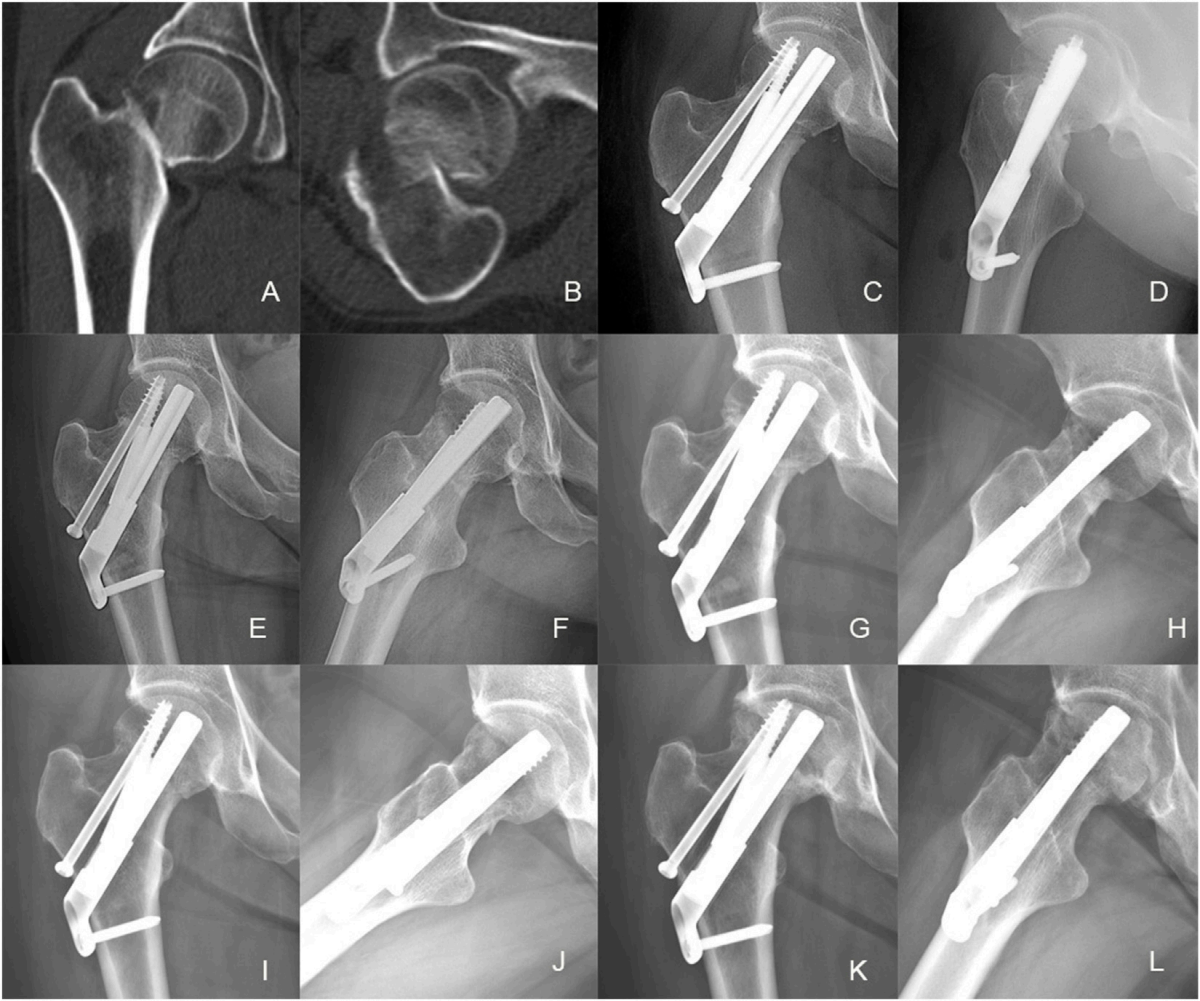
Case presentation: a 46 years old woman with right femoral neck fracture fixed with FNS. (A,B) Preoperative CT scan showed the right FNF with posteromedial cortex fracture. (C,D) The anteroposterior and lateral X-ray with FNS 1 day after surgery; (E,F) The anteroposterior and lateral X-rays 1 month after surgery; (G,H) The anteroposterior and lateral X-rays 3 months after surgery; (I,J) The anteroposterior and lateral X-rays 6 months after surgery; (K,L) The anteroposterior and lateral X-rays 12 months after surgery.
